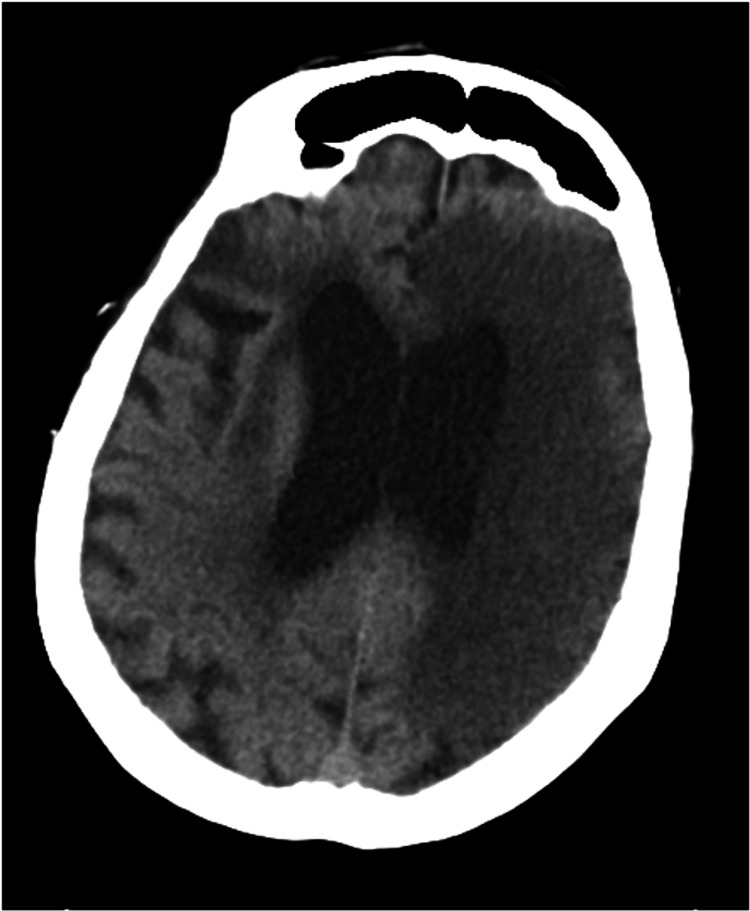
Computed tomography (CT) scan demonstrating a large hypodense area on the left, corresponding to a middle cerebral artery (MCA) infarct
